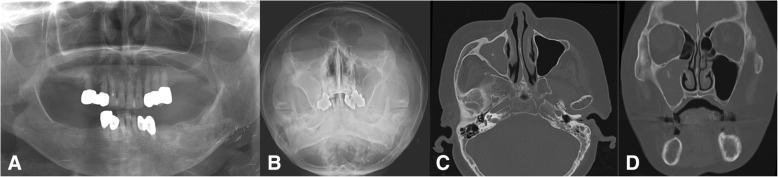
Chronic maxillary sinusitis originating from the right second premolar, the crown of which was analyzed or continuous denture loading. Preoperative panoramic view (a), Water’s view (b), and axial CT scan view showing radiopacity in the middle of the sinusitis suspicious of fungal ball (c), and coronal CT scan view showing definite fungal maxillary sinusitis with ostium obstruction (d)
